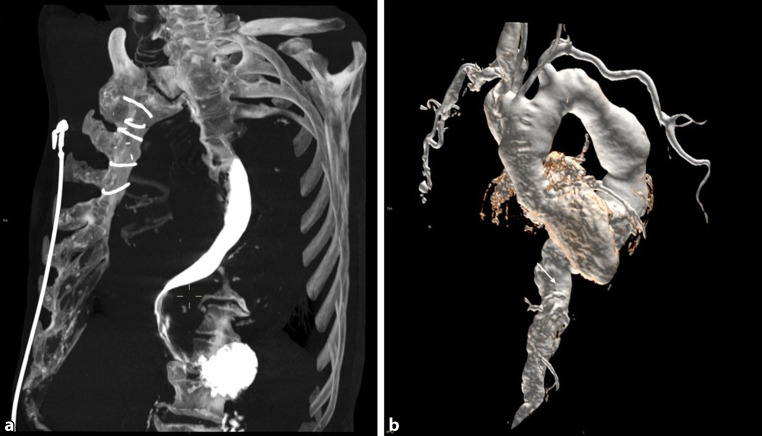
a CT scan after oral contrast administration with maximum intensity projection reconstruction showing esophageal compression. b Magnetic resonance angiography after oral administration of gadolinium-based contrast, showing the whole anatomy of the aortic aneurysm lumen with a virtual rendering technique
